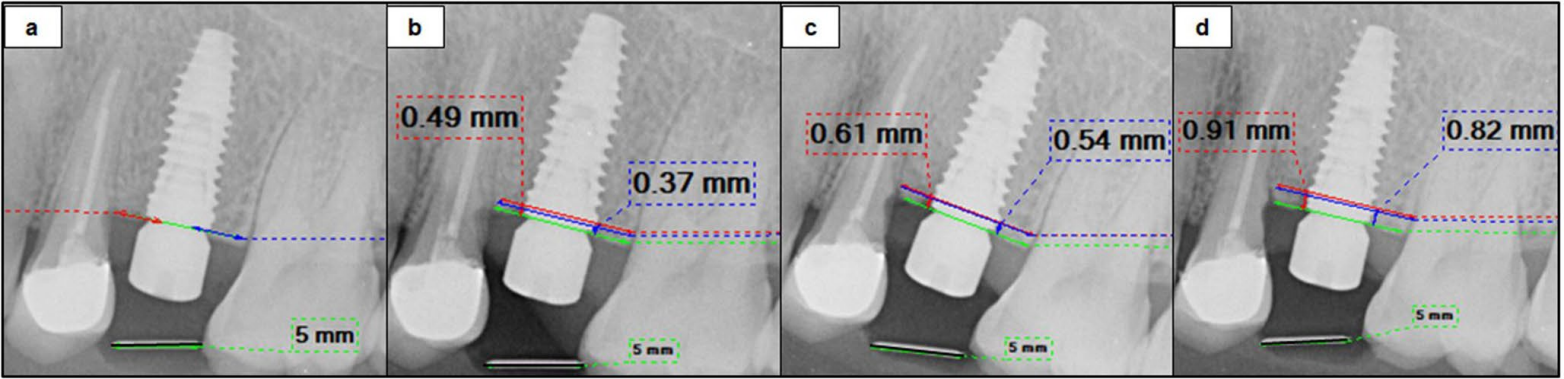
Radiographic assessment of crestal bone loss from implant platform (green line) to the first visible bone-to-implant contact; mesially (red line) and distally (blue line), at each time point (a: post-insertion, b: 3 months, c: 6 months post-loading and d: 12 months post-loading)
